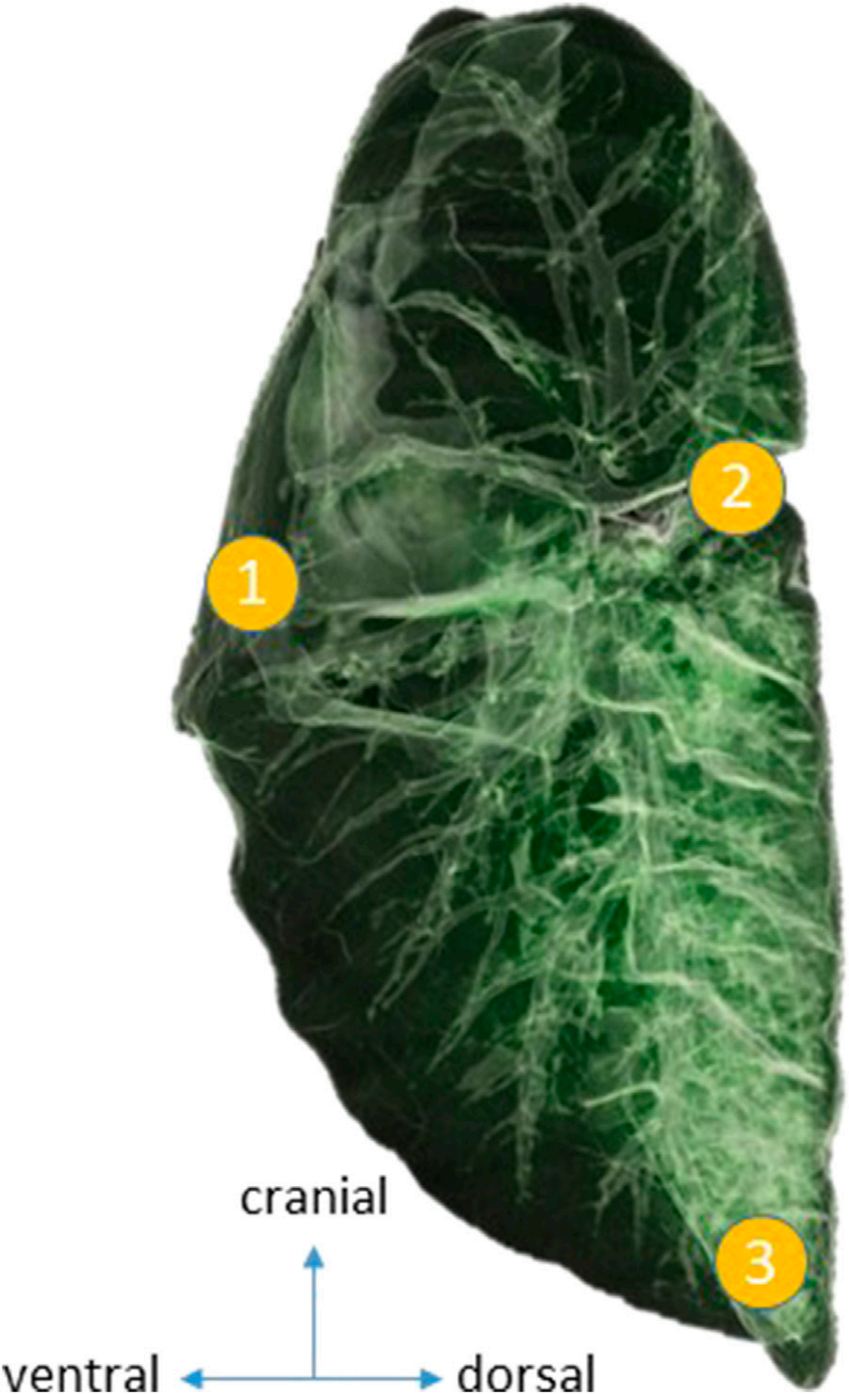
Position of the intrapleural pressure sensors. To measure regional pleural pressure, three pressure sensors were custom-made, calibrated, and placed via video-assisted thoracoscopy. The sensors were attached to the parietal pleura by staples in the following regions of the left hemithorax: 1) ventral (4–5th rib parasternal); 2) dorsal (4–5th rib paravertebral); and 3) caudal (8–9th rib paravertebral).
